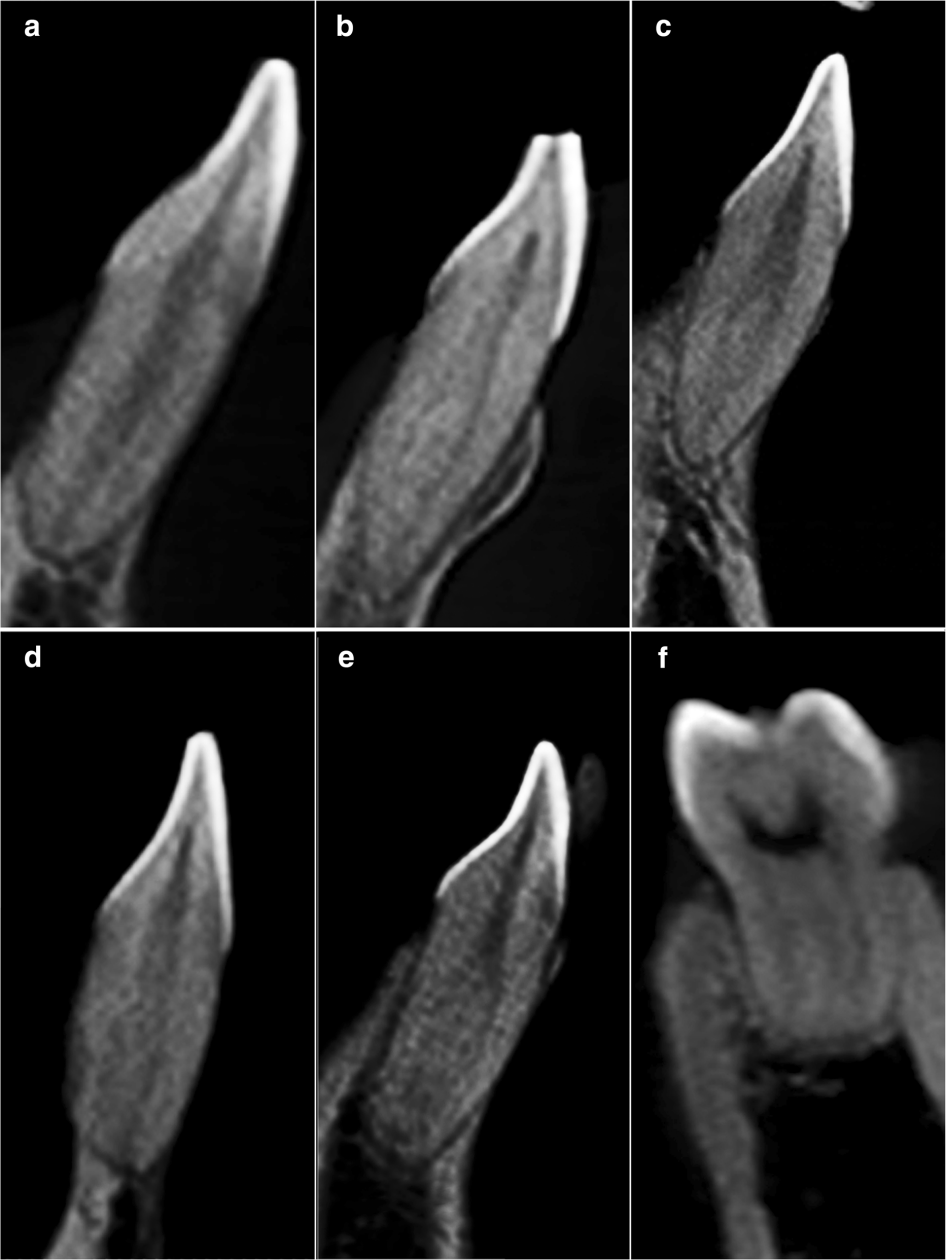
CBCT images of mandibular teeth in custom planes (reconstructed based on axial, sagittal and coronal planes) based on Vertucci’s classification. a Type I – left mandibular central incisor (b) Type II – right mandibular central incisor (c) Type III – left mandibular lateral incisor (d) Type IV – left mandibular central incisor (e) Type V – right mandibular left lateral incisor (f) Type VIII – left mandibular first molar
